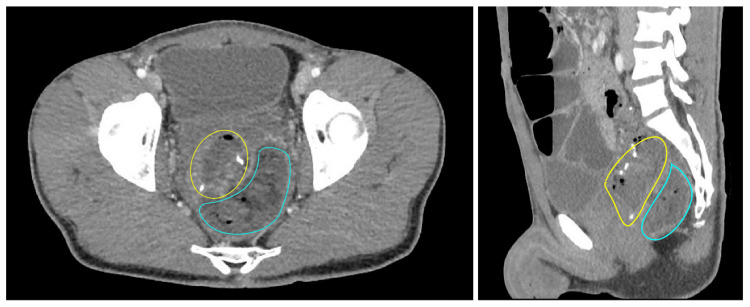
Axial and sagittal views of IPAA with staple lines outlined in yellow with posterior abscess/leak cavity outlined in blue on CT abdomen pelvis with contrast.
